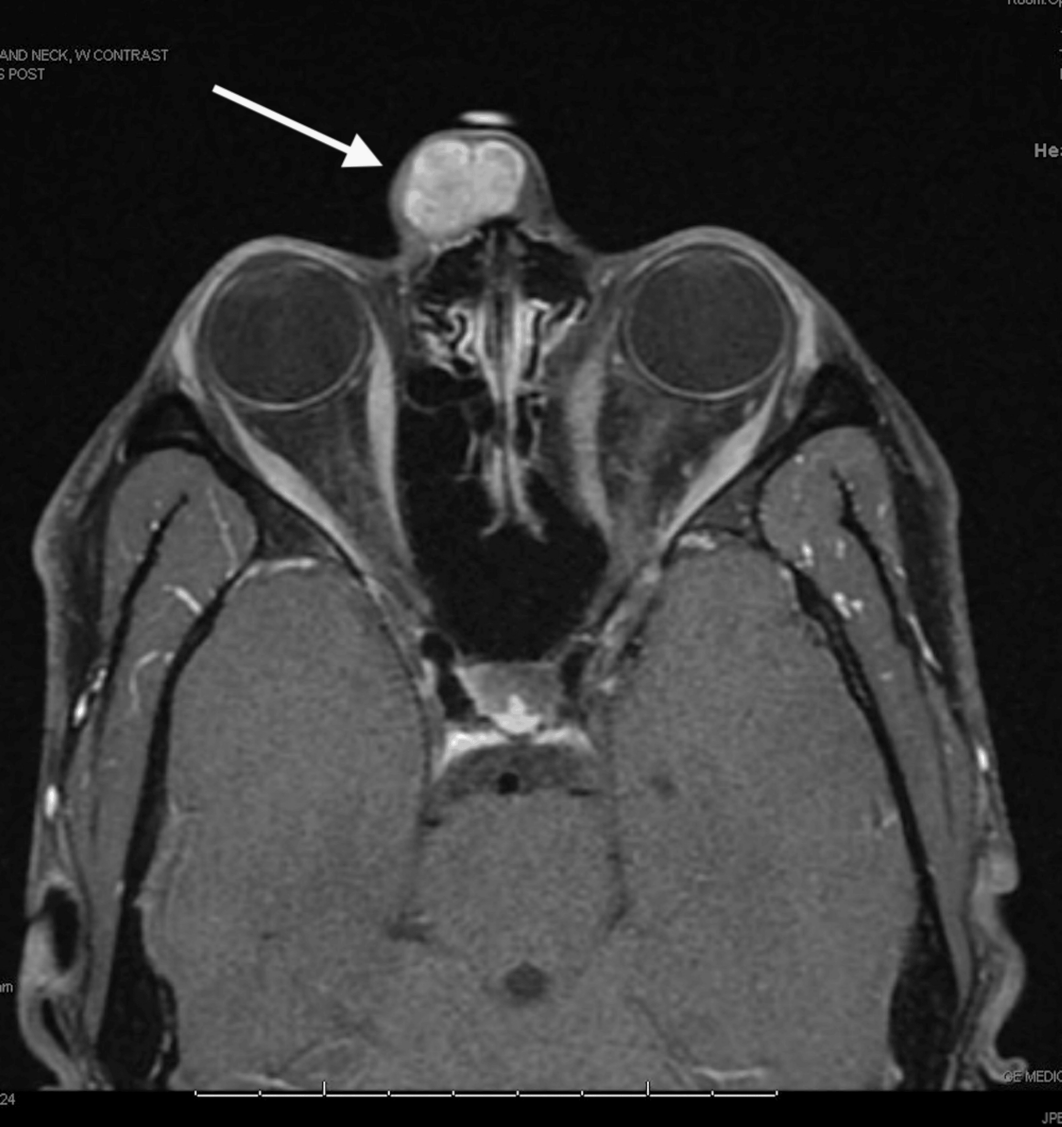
Preoperative MRI (post-contrast T1-weighted) Preoperative MRI: axial cut of a post-contrast T1-weighted image with an arrow demonstrating a contrast-enhancing mass within the glabellar region MRI: Magnetic resonance imaging
